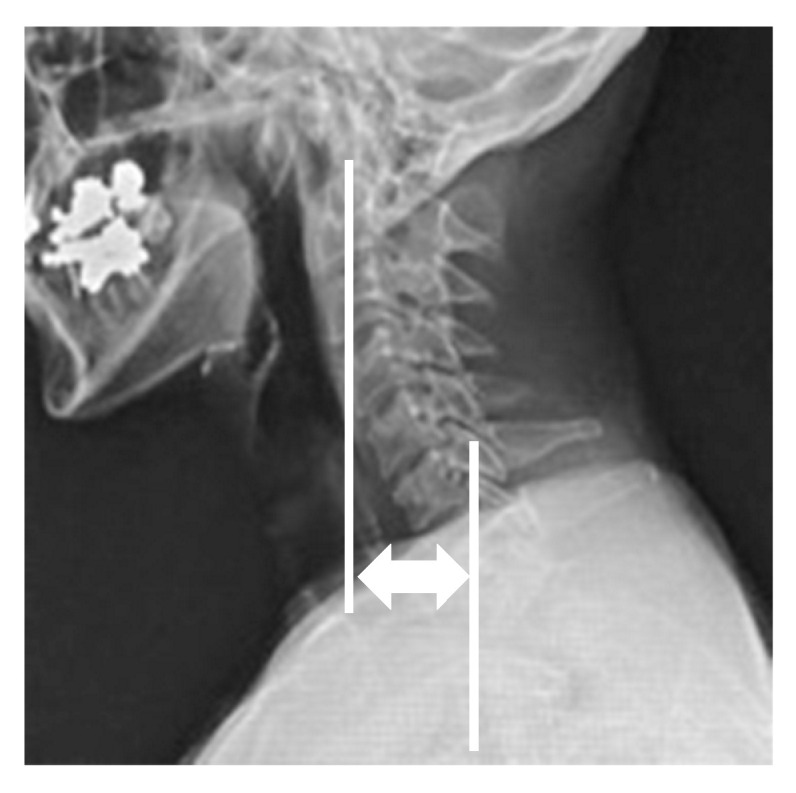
Radiographic parameters; C2–7 sagittal vertical axis.
